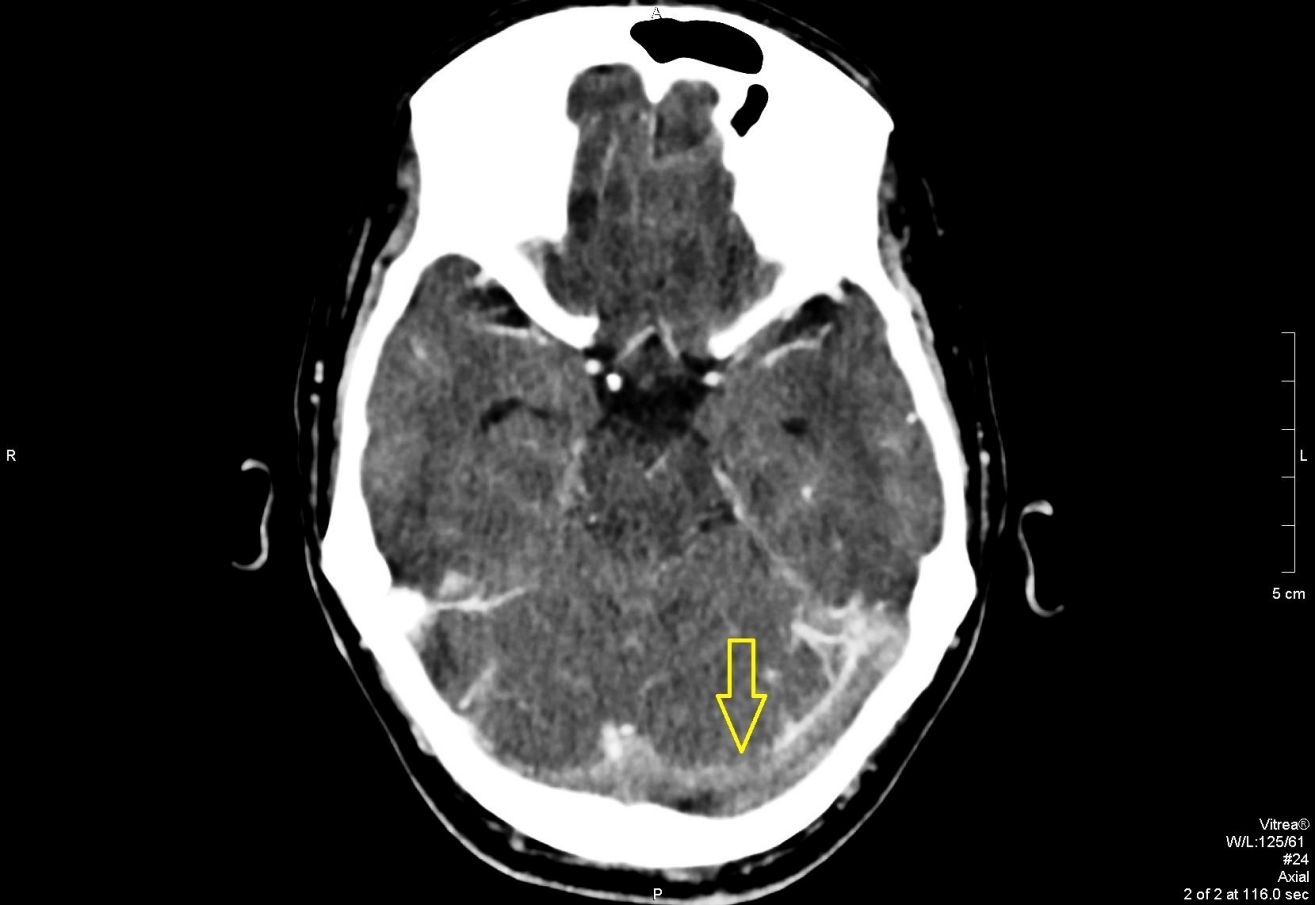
Axial view on contrast CT scan showing transverse sinus blockage CT: computed tomography
